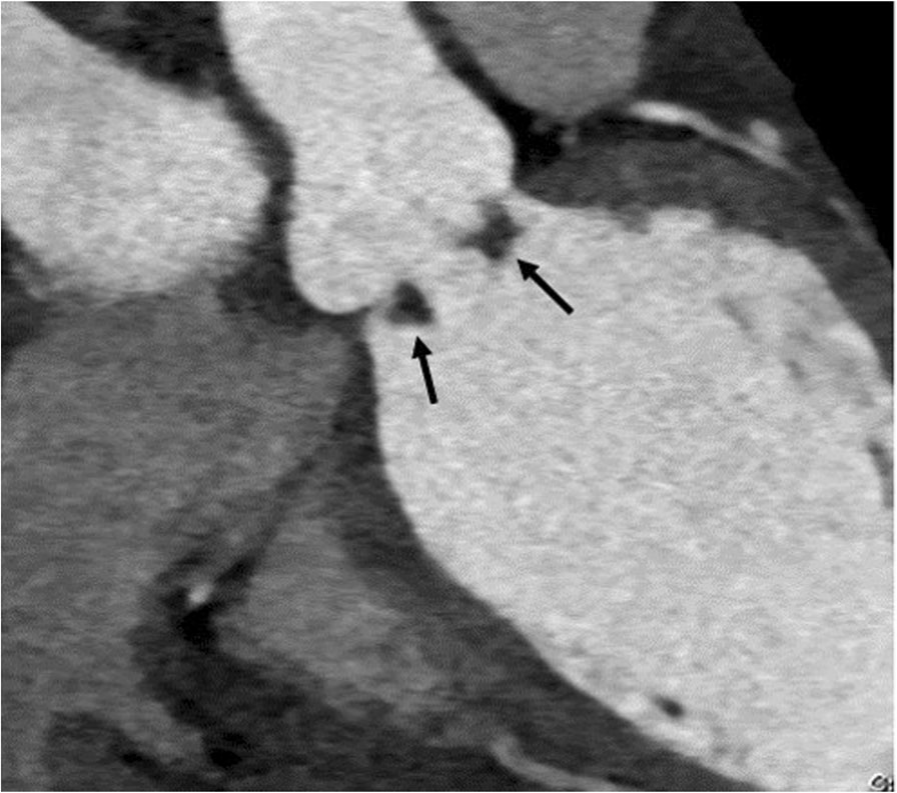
A 34-year-old man with rheumatic valve disease. On cardiac CTA showing nodular low-density lesions at aortic valve (arrow). Clinically, vegetation of rheumatic valve disease was suspected. In the operation, vegetation was noted in the rheumatic pathology of the aortic valve
